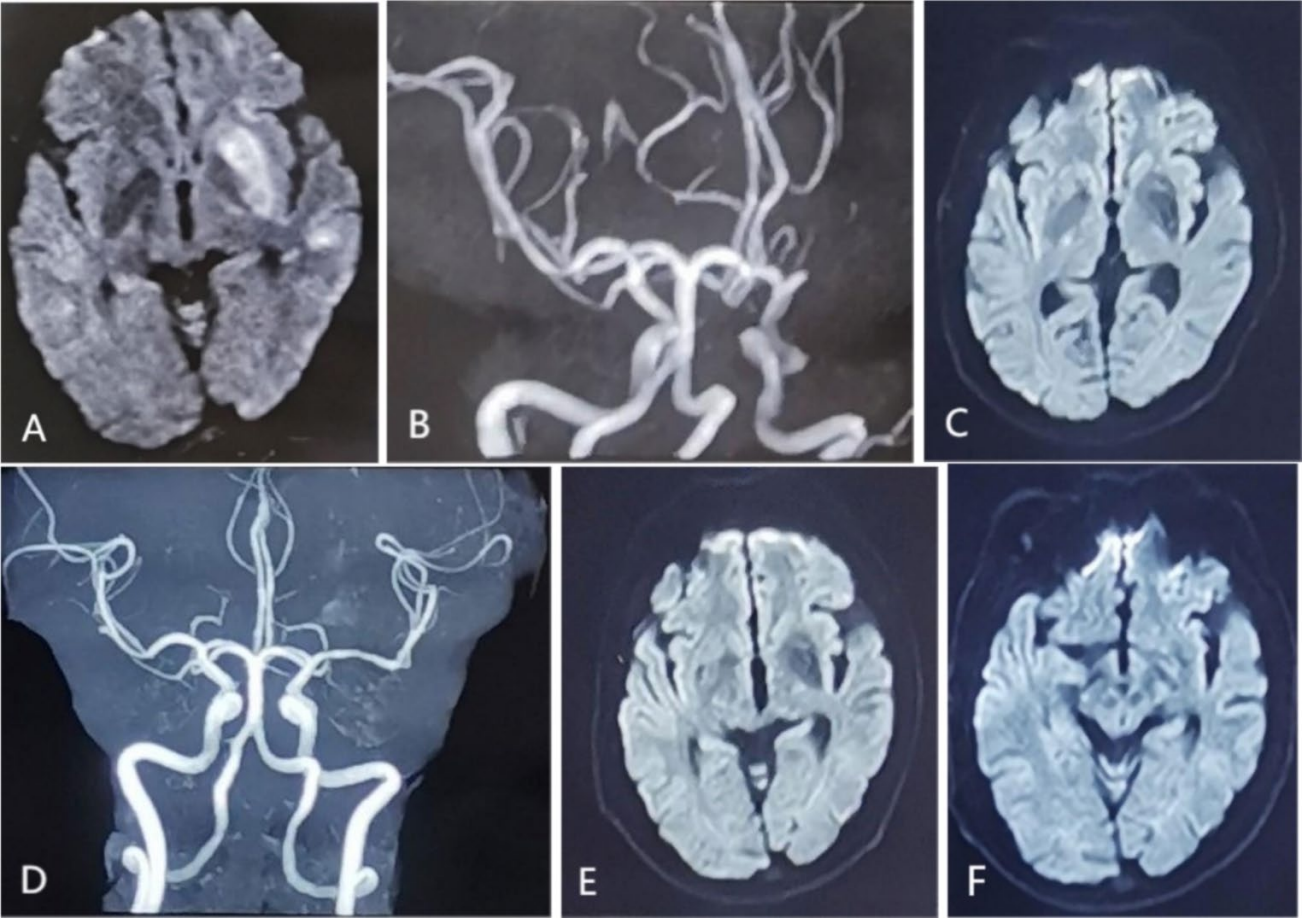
Magnetic resonance during the course of the disease. A: DWI showed left basal ganglia and temporal lobe hypersignal shadow (April 3, 2023); B: MRA displayed the M1 segment occlusion of the left middle cerebral artery(April 3, 2023); C: DWI revealed no abnormal high signal(July 21, 2023); D: MRA showed recanalization of the left middle cerebral artery occlusion segment; E: DWI revealed no abnormal high signal(December 5, 2023); F: DWI revealed no abnormal high signal(April 11, 2024)
